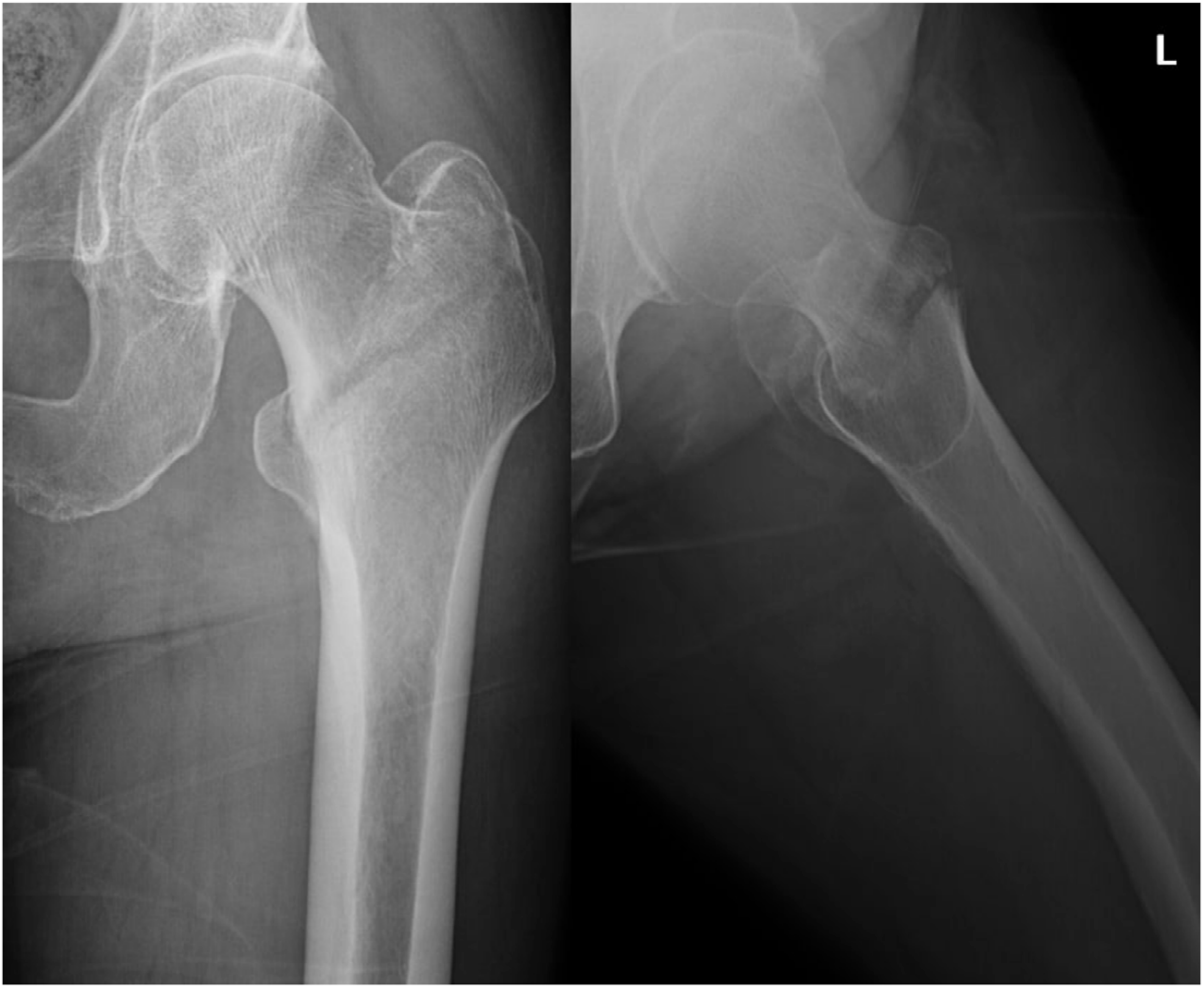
Preoperative radiographs.
